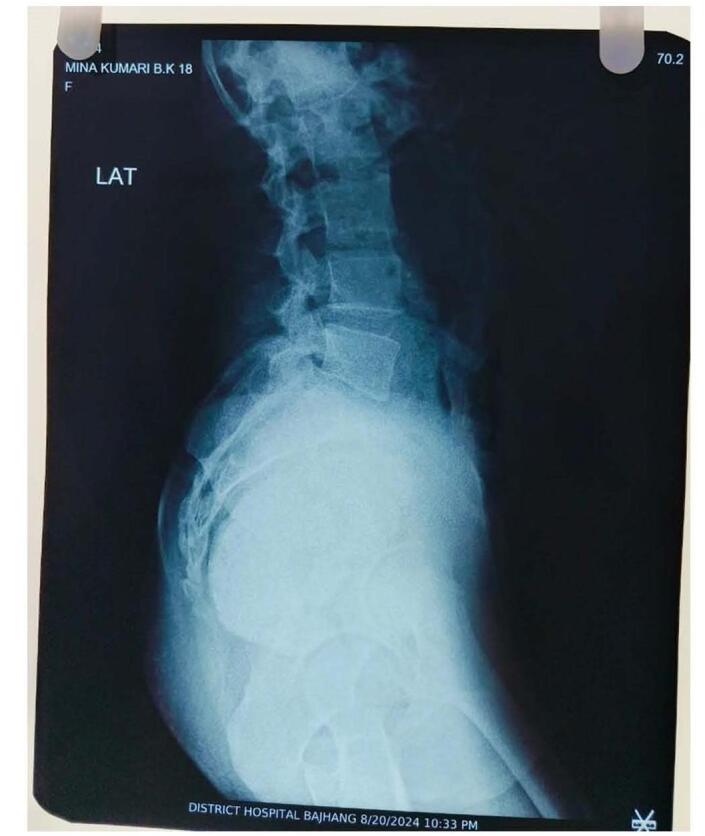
X-ray lateral view showing the 6 × 5 cm radiopacity lying in front of the sacral bone.
